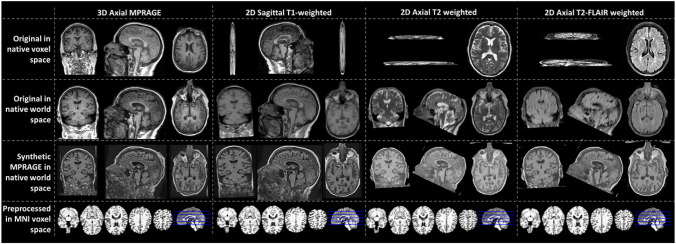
Predicted MPRAGEs for three of the five modalities included in this study. The data belongs to a single participant. The top row shows selected slices in the slice, phase-encoding and readout directions (left, right top and right bottom, respectively). The bottom row shows the bottommost (z = −27 mm, slice 46), topmost (z = 53 mm, slice 126), and three intermediate slices (z = −7, 13, and 33mm, slices 66, 86 and 106, respectively) of the 80 slices used for brain age prediction. To show images in world space, trilinear interpolation was used. FLAIR: Fluid attenuated inversion recovery.
